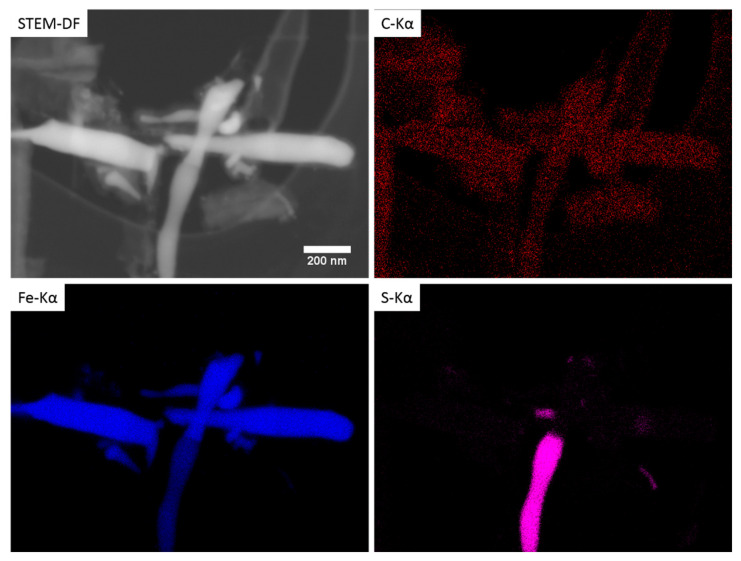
Elemental X-ray map of SCNA8 sample in STEM mode at E0 = 20 kV.
